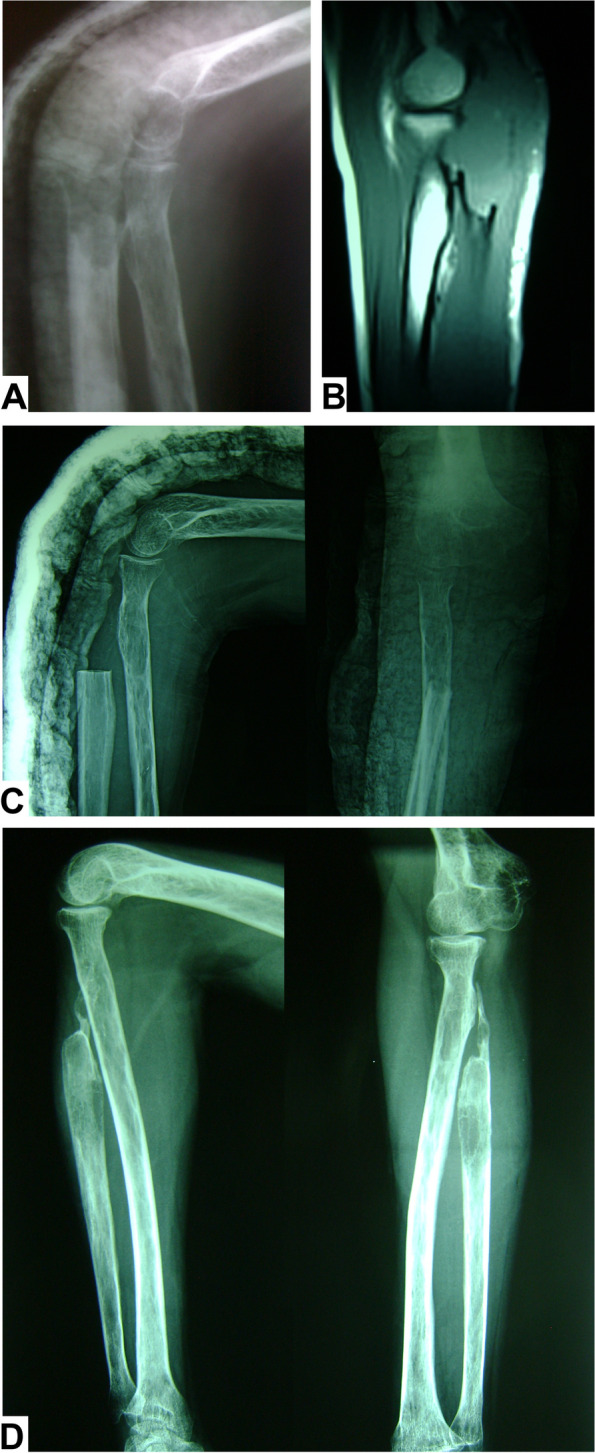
A 20-year-old male with Ewing's sarcoma of the proximal ulna treated by excision arthroplasty. A Preoperative X-ray lateral view. B Preoperative MRI sagittal view. C Immediate postoperative X-rays anteroposterior and lateral views. D Three-year follow-up X-rays anteroposterior and lateral views
